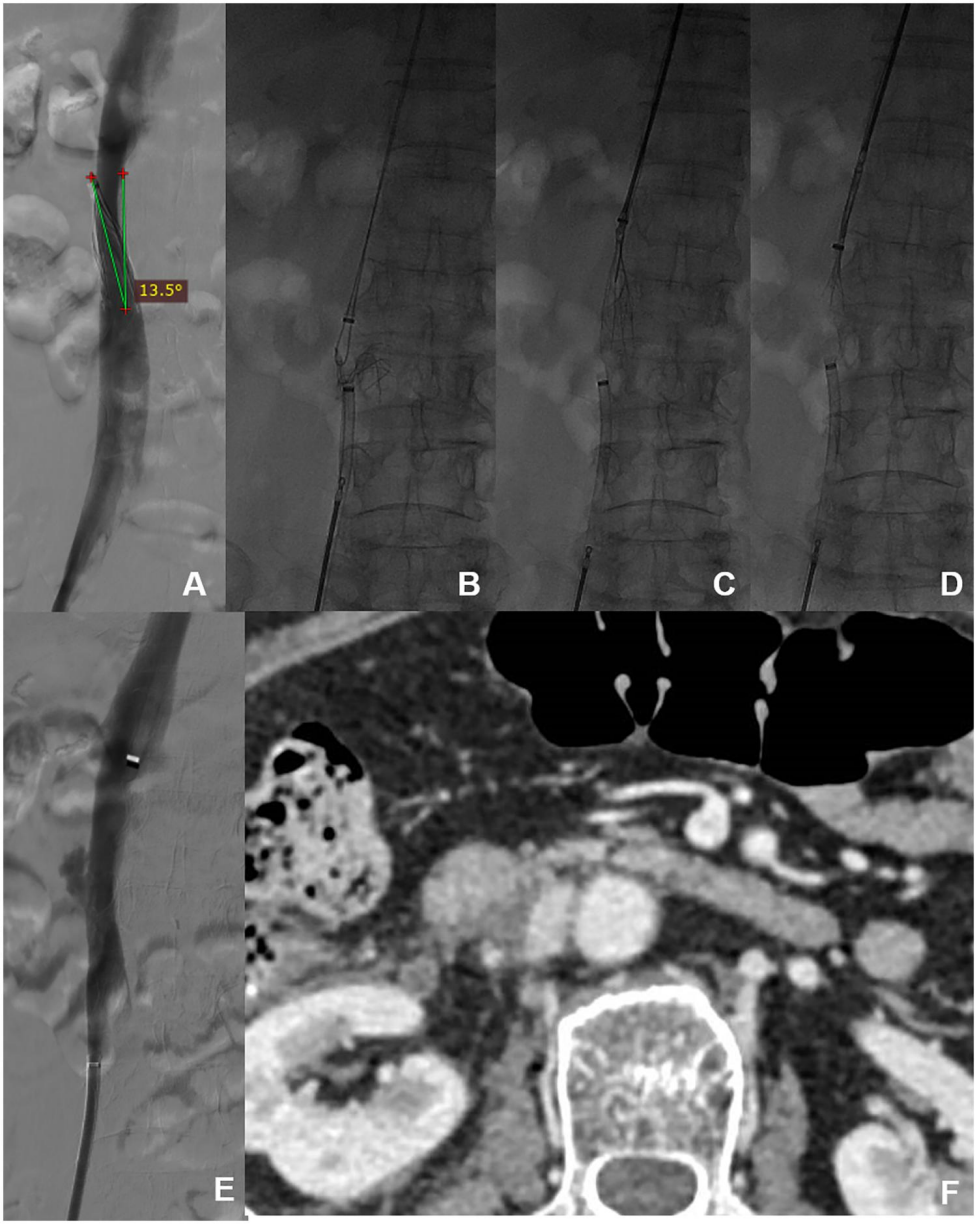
Complications during the filter's retraction hook capture technique of pull-assisted method for tilted wall-attached conical filter. (A) The conical filter has a tilt angle of 13.5°. (B) The distal end is pulled with a snare, and advanced retrieval techniques are attempted at the proximal end. (C) The proximal end uses forceps to capture the filter retrieval hook. (D) The snare successfully retrieves the filter. (E) IVC damage is observed. (F) Postoperative enhanced CT scan of the IVC one week after Interventional surgery.
